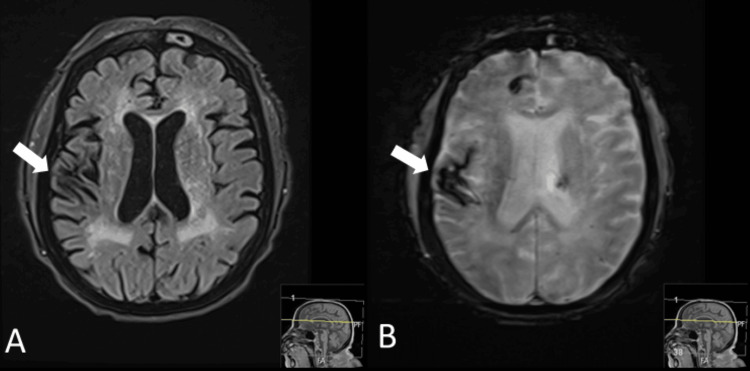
Corresponding MRI findings of the right temporoparietal lobe lesion on T2-weighted and SWI sequences A: linear T2 hypointensity in the right temporoparietal region consistent with encephalomalacia (arrow); B: susceptibility artifact in the same region on SWI indicates chronic hemosiderin deposition (arrow), consistent with prior hemorrhage. SWI: susceptibility-weighted imaging
